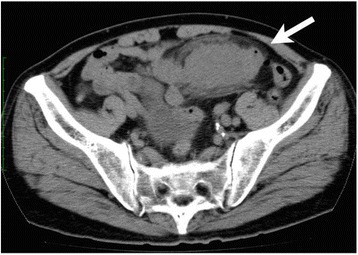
Computed tomography. Abdominal computed tomography showing an intra-abdominal abscess adjacent to the small intestine
